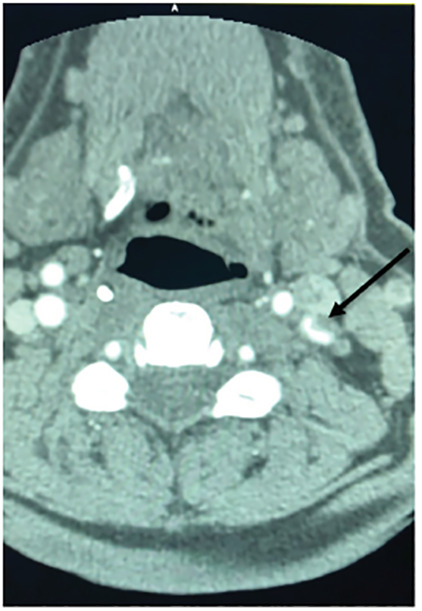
Computed tomography demonstrating dissection of the left internal carotid artery depicting “crescent sign” (arrow).
